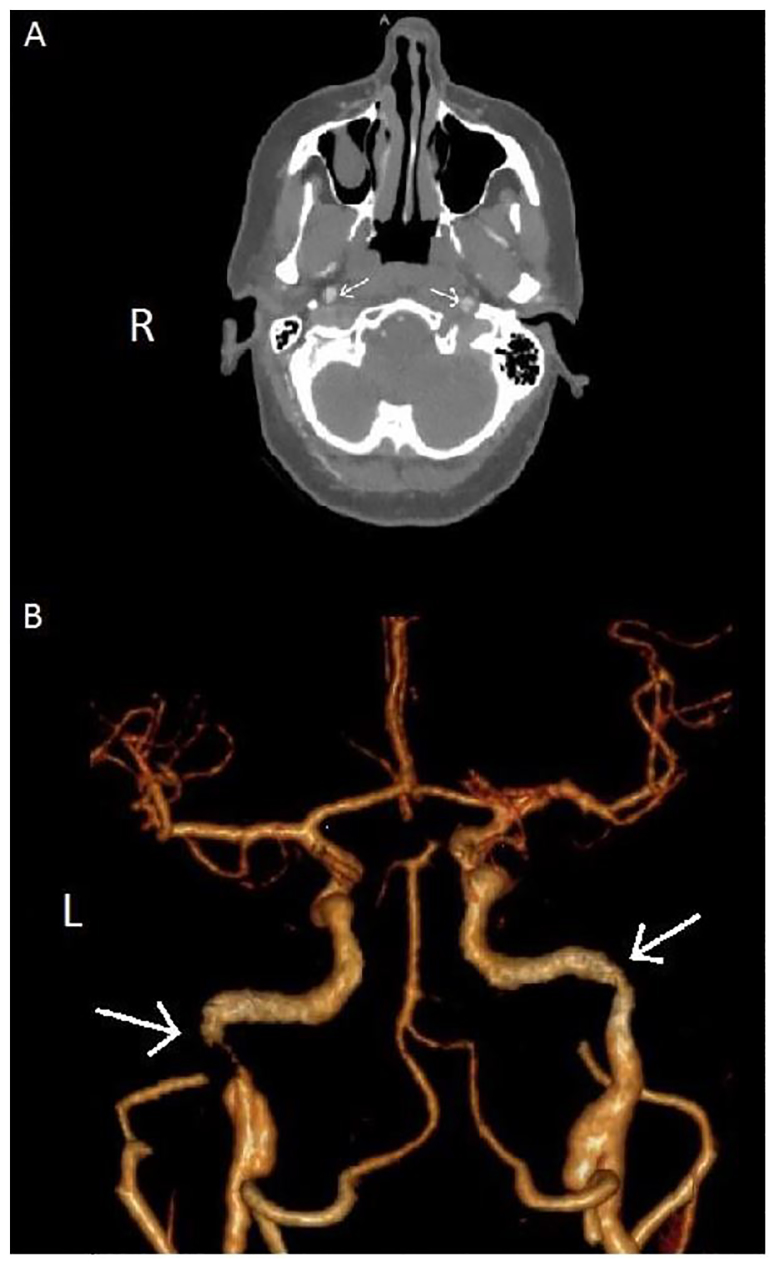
A. Axial CT angiography. Arrows mark intimal flaps in right and left internal carotid arteries. B. 3D reconstruction of CT angiography of the brain with luminal narrowing of bilateral internal carotid arteries marked by arrows. *CT,* computed tomography
